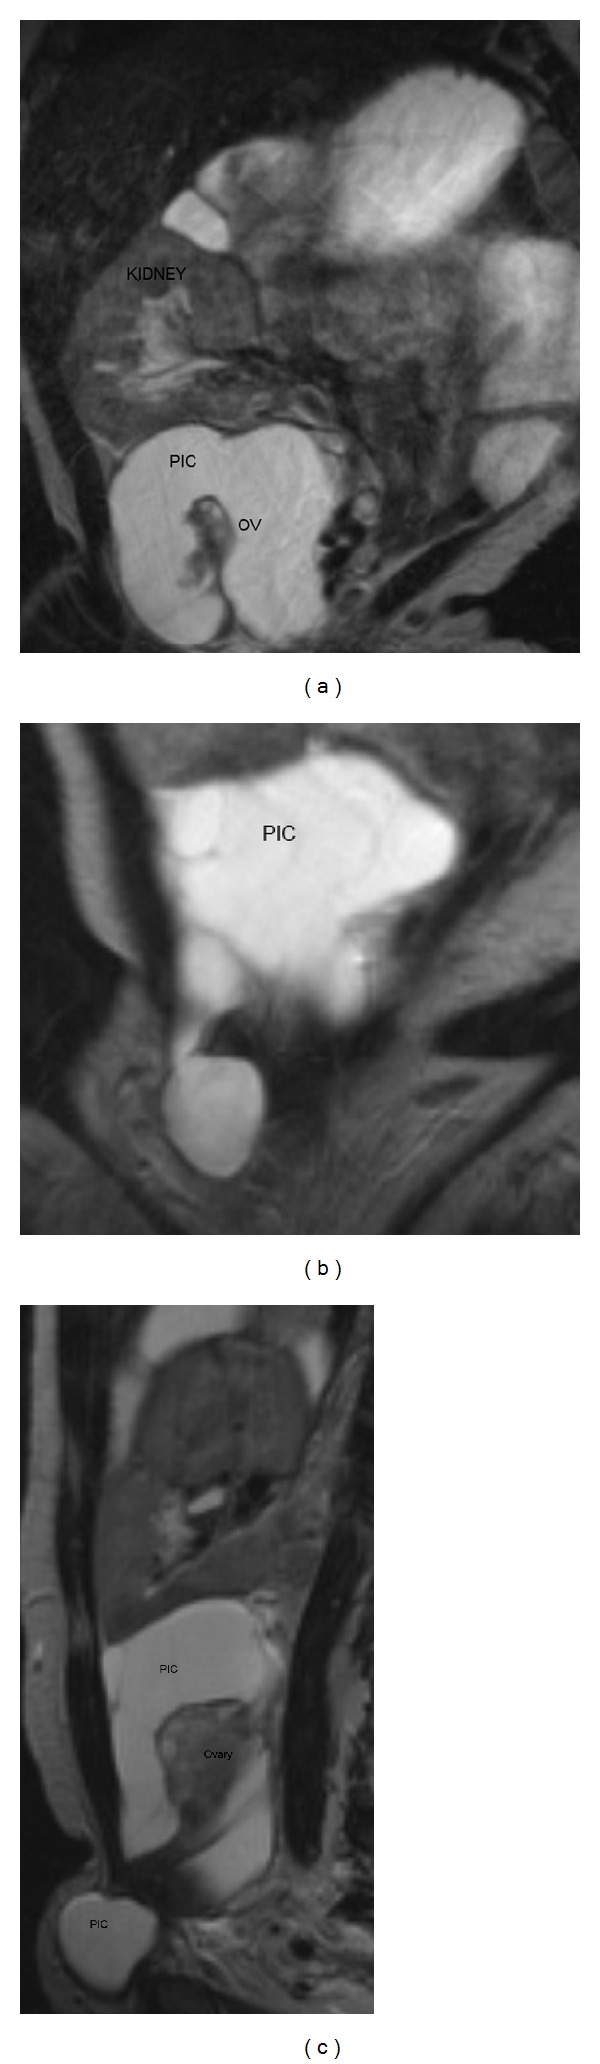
(a) Coronal T2 MR image demonstrating a cystic mass (PIC) containing ovarian tissue (OV) in the right adnexal region, extending into the inguinal canal. A transplanted right pelvic kidney is noted. (b) Coronal T2 MR image of peritoneal inclusion cyst (PIC) with extension to the right inguinal canal. Note “spider web” septations. (c) Sagittal T2 MR image of peritoneal inclusion cyst (PIC) with “spider web septations” containing ovarian tissue, with extension to the right inguinal canal.
